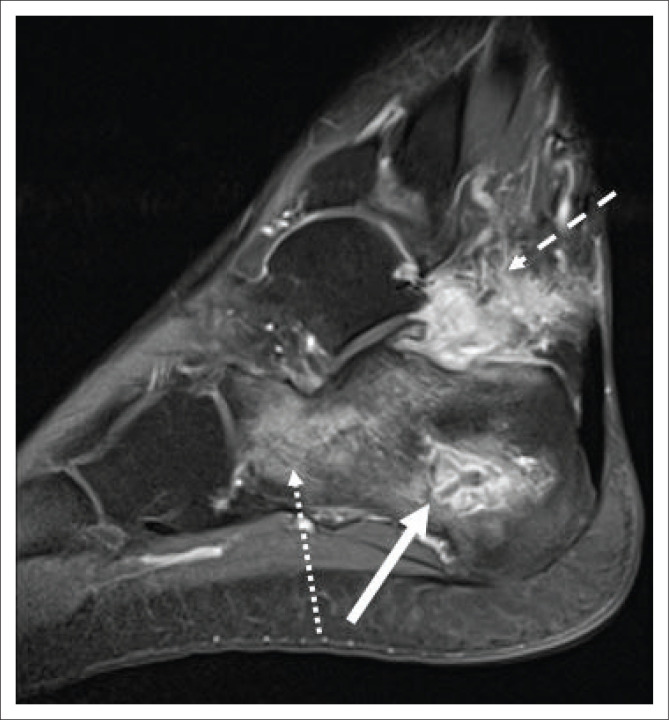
A 23-year-old male, human immunodeficiency virus status unknown, presented with left heel pain and a draining sinus. Sagittal T1-weighted fat sat, post-contrasted magnetic resonance imaging of the left hind foot demonstrates a small rim-enhancing collection in the calcaneus (solid white arrow) with enhancing inflammatory changes in the remainder of the calcaneus (white arrow with dotted line) and surrounding soft tissues (white arrow with dashed line). A draining sinus was demonstrated on T2W sequences. Tuberculosis was confirmed on Gene Xpert of calcaneal tissue.
